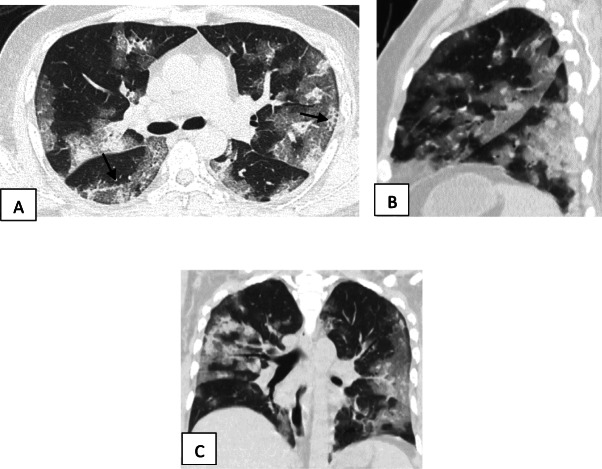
Unenhanced chest CT of 52-year-old male patient with COVID-19. a Axial lung window image shows bilateral subpleural and broncho-vascular areas of ground glassing opacity, consolidation, crazy paving, and fibrosis [single head arrows]. b Sagittal and c coronal reformatted images. CT score is 19
